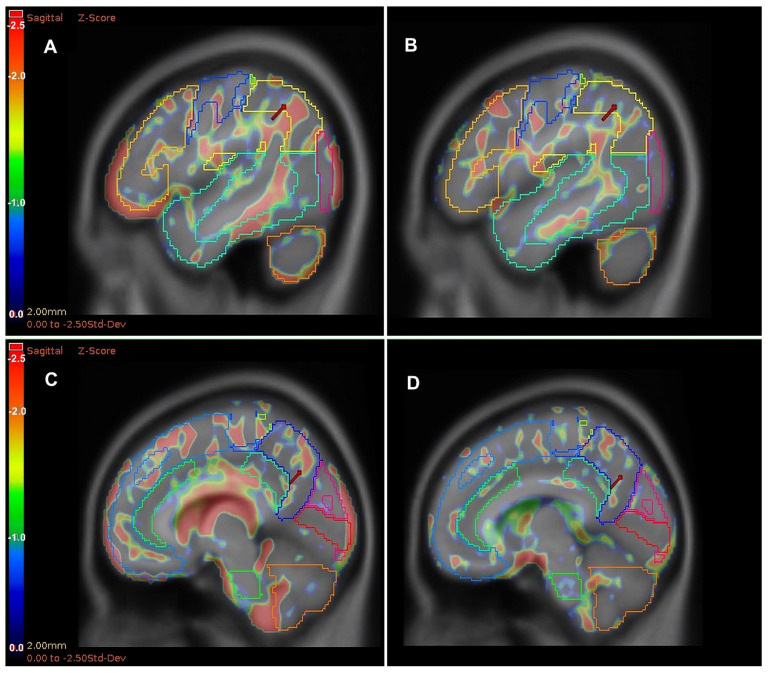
Visual presentation of hypometabolic brain areas in the FDG PET-CT scan in two patients with different PLRs. (A): Left parietal inferior lobulus delineated with yellow and marked with red arrow, uptake ratio = 0.93, Z-Score = −1.98. (B): Left parietal inferior lobulus delineated with yellow and marked with red arrow, uptake ratio = 1.74, Z-Score = 0.58. (C): Left precuneus delineated in navy blue and marked with red arrow, uptake ratio = 1.74, Z-Score = −0.59. (D): Left precuneus marked with red arrow, uptake ratio = 1.86, Z-Score = 0.40. For A and C, PLR = 98.3 and MoCA abstraction = 0/2; for B and D, PLR = 168.8 and MoCA abstraction = 2/2.
